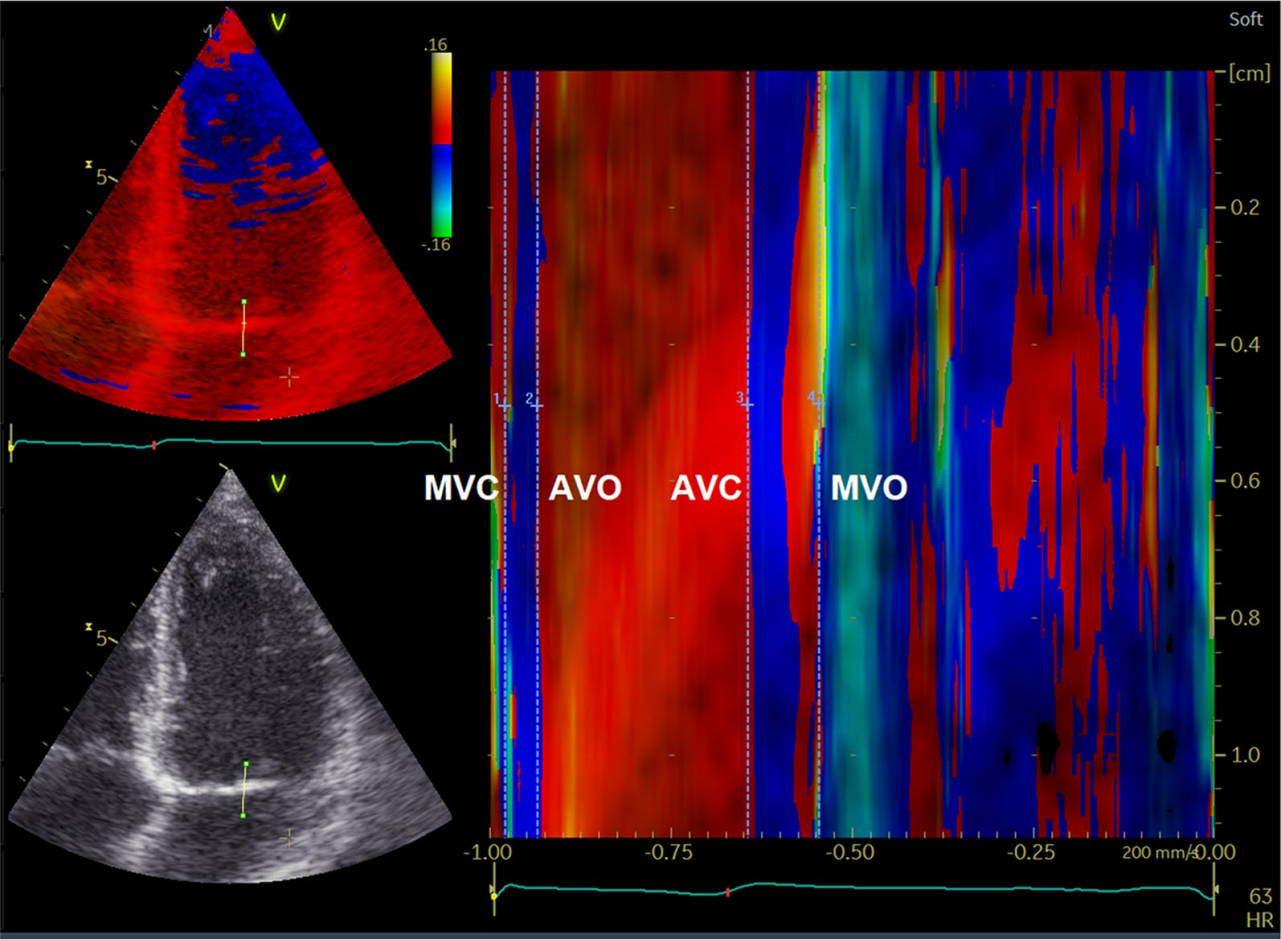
The cardiac time intervals assessed by color tissue Doppler imaging (TDI) M-mode through the mitral leaflet. *AVO* aortic valve opening, *AVC* aortic valve closing, *MVC* mitral valve closing, *MVO* mitral valve opening
